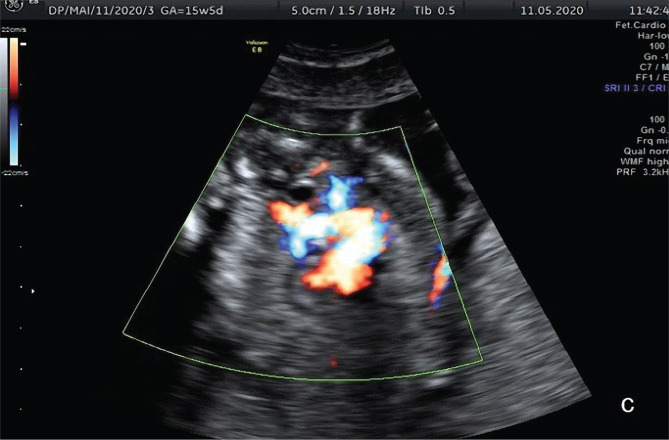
2D and color Doppler ultrasound diagnosis of micrognathia (a), double bubble sign strongly indicative for duodenal atresia (b) and common truncus arteriosus (c).
